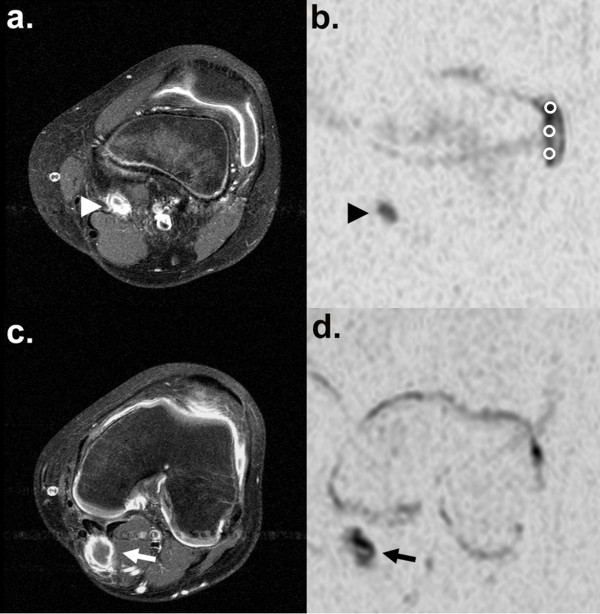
Diffusion-weighted imaging in a patient with gonarthritis and a popliteal cyst. Arthritis of the left knee in a 14-year-old boy with JIA. Post-contrast T1W (a) and DWI b = 1000 with inverted gray scale (b) both show synovial signal changes, layered signal of the parapatellar lateral recessus in the presence of effusion and reactive popliteal lymphadenopathy (arrow head). With 6 averages and 2 min 50 s scan time, DWI signal and image quality are substantially improved, as compared to Figure 4. A caudal cross-section of the same knee joint depics a Baker cyst in the medial popliteal fossa, also showing signs of synovitis (arrow) on both post-contrast T1W (c) and DWI (d). ADC measurement with three small circular regions of interest (ROI) is demonstrated in (b).
